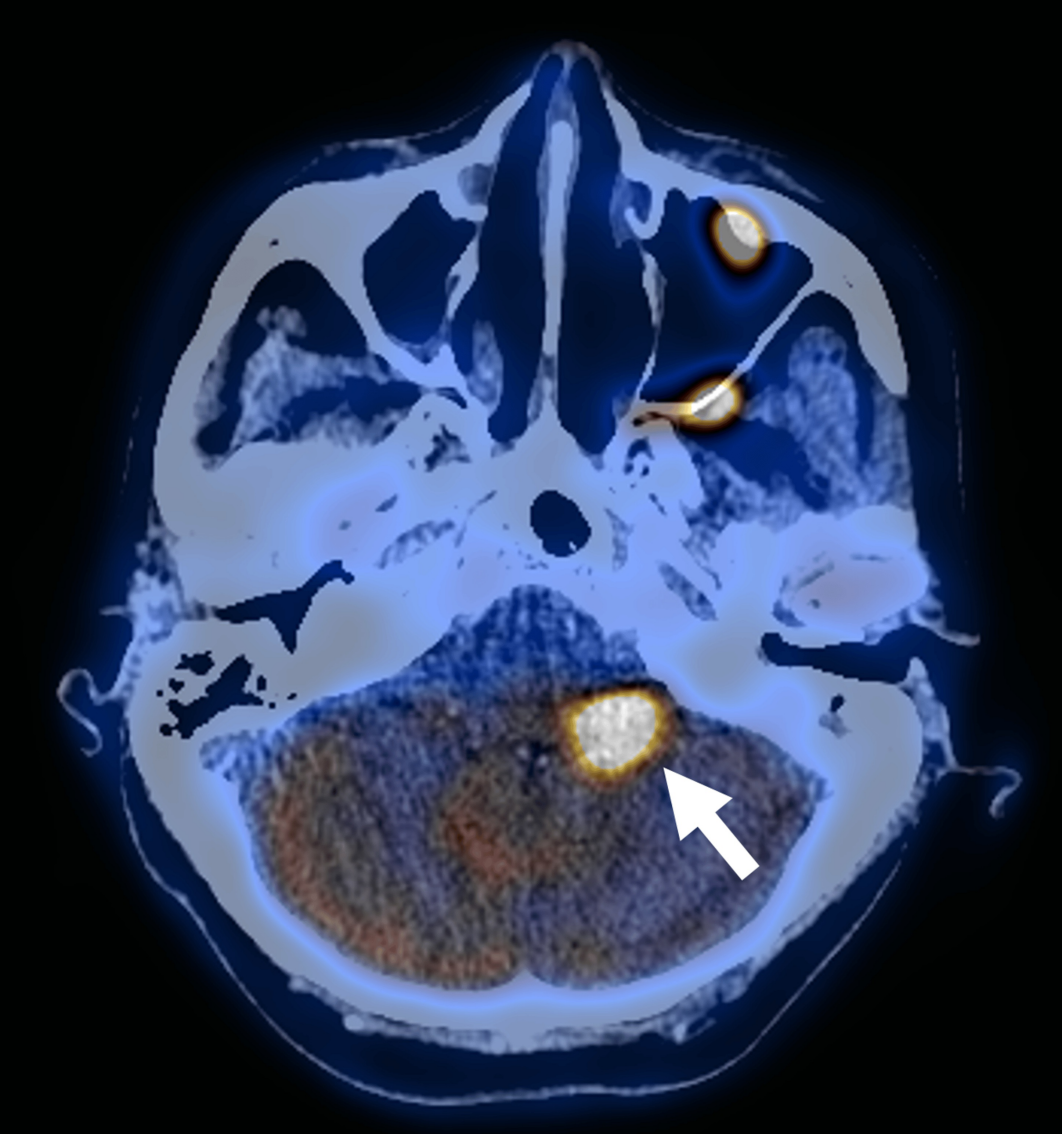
PET/CT fusion axial image showing focal hypermetabolism involving the left side of pons
